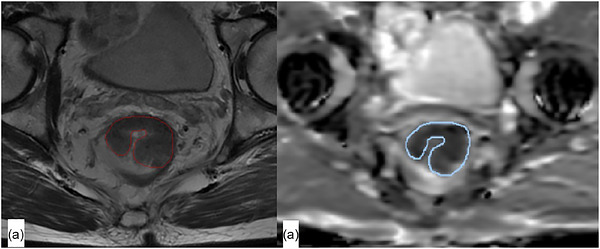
Imaging sequences and tumor volume calculation. (a) FOV and axial T2WI sequences; (b) Axial apparent diffusion coefficient (ADC) sequence. The left anterior half of the intestinal wall was thickened. The tumor infiltrated the proper muscle layer and involved the mesorectum, with evidence of extramural venous invasion (EMVI). The regions of interest (ROIs) were delineated on a para‐axial high‐resolution T2‐weighted sequence and adjusted with reference to diffusion‐weighted imaging (DWI)/ADC sequences. The tumor boundaries were traced layer by layer, and the tumor volume (in cm^3^) was automatically calculated using TPS software.
